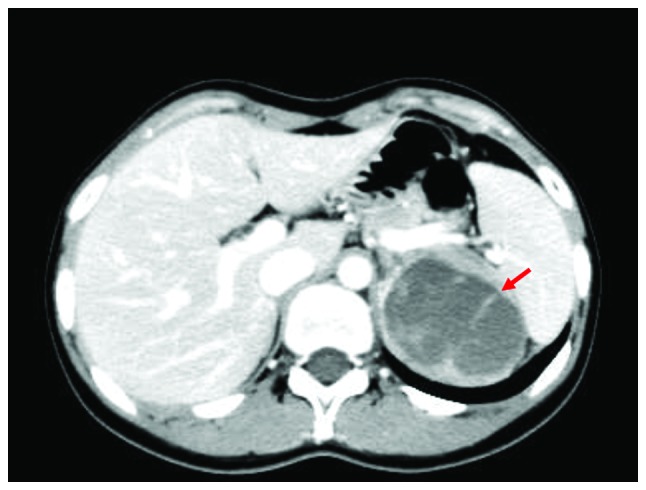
Computed tomography scan showing an adrenal mass suspected to be a possible adrenal tumor.
